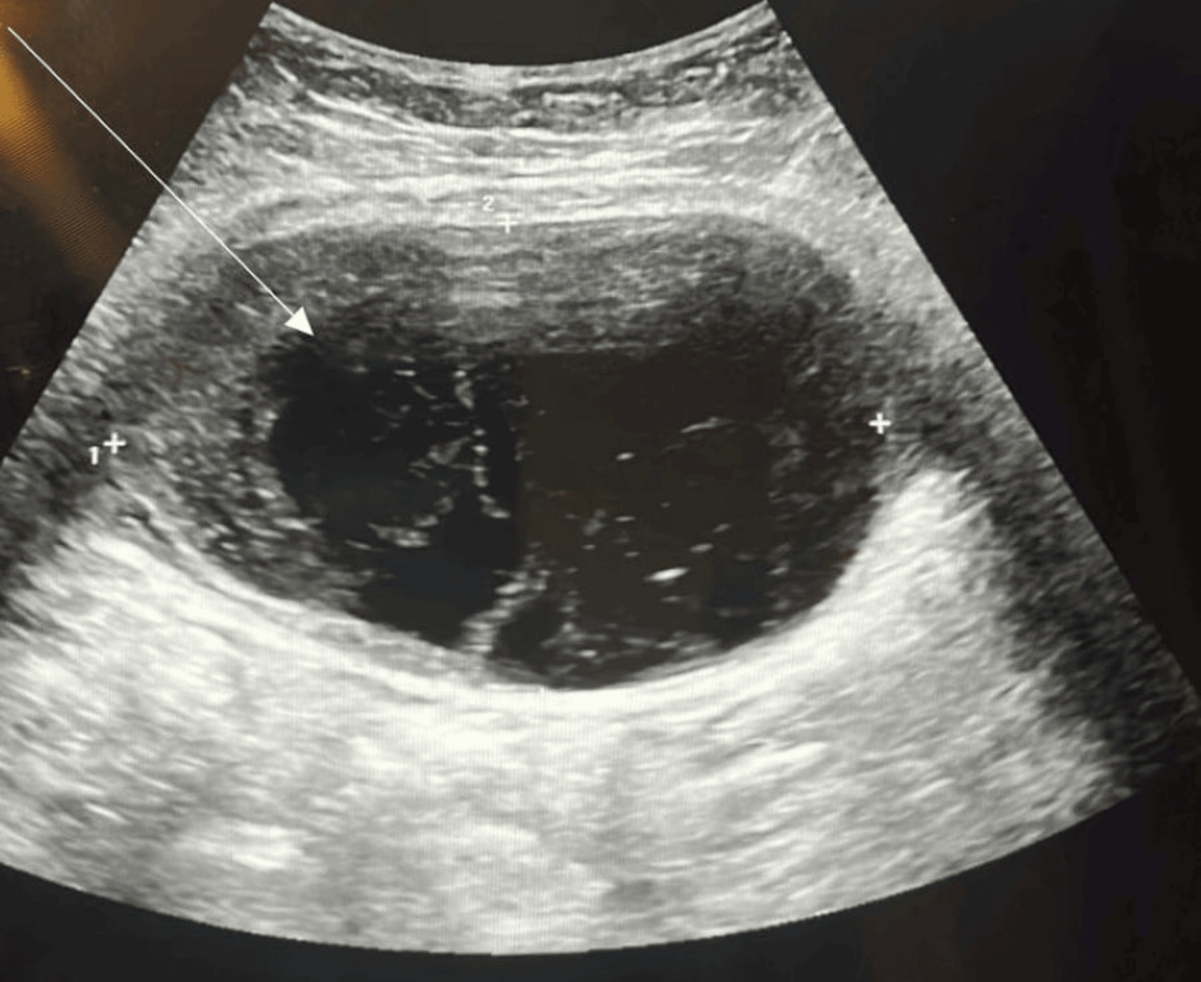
Ultrasound scan image at two weeks post-op (arrow indicates the haematoma).
